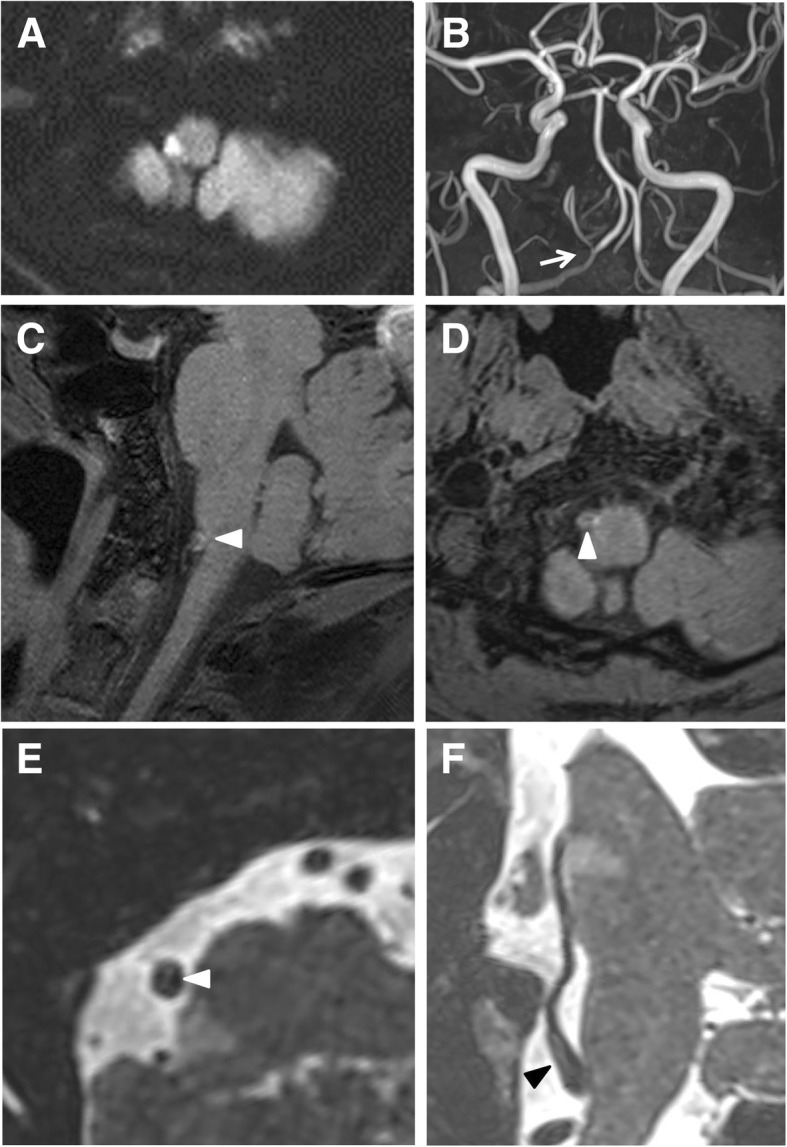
Brain magnetic resonance image (MRI), time-of-flight MR angiography (TOF-MRA), and high-resolution vessel wall MRI (HR vw-MRI) in case 1. Diffusion weighted image (DWI) shows acute infarction in the right lateral medulla (a). Stenosis of the right proximal posterior inferior cerebellar artery (PICA) is seen on TOF-MRA (arrow) (b). Three-dimensional (3D) sagittal T1 weighted HR vw-MRI reveals a hyperintense intramural hematoma in the right proximal PICA (white arrow head) (c). 3D oblique axial T1 weighted HR vw-MRI shows a linear low signal intensity within the right proximal PICA (white arrow head) (d). 3D oblique axial T2 weighted HR vw-MRI shows a linear high signal intensity within the right proximal PICA (white arrow head) (e). Curved multiplanar reconstruction (MPR) image reconstructed from 3D T2 weighted HR vw-MRI shows an entire intimal flap clearly in one image (black arrowhead) (f)
